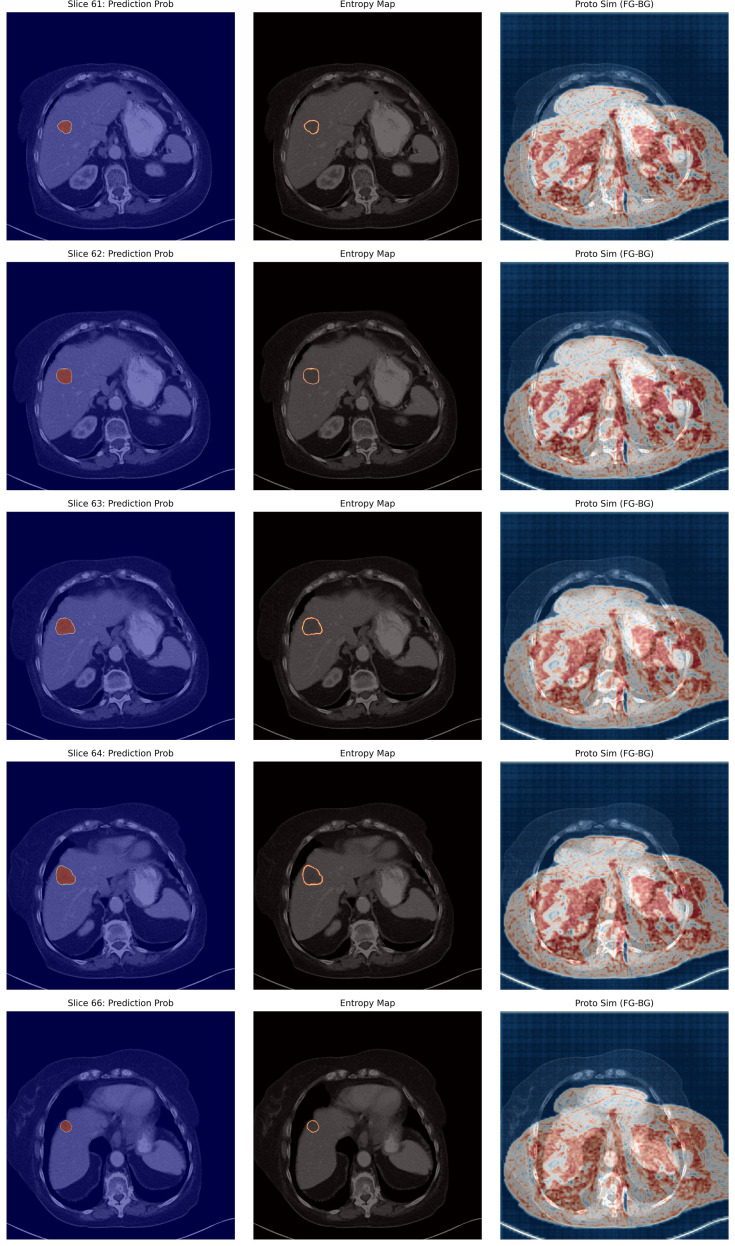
Entropy and prototype-response visualization for the same five slices shown in Figure 2. For each slice, the figure presents the CT image, the entropy map, the foreground prototype similarity map, and the background prototype similarity map. High-uncertainty responses are mainly concentrated around ambiguous lesion boundaries, while the foreground prototype similarity is concentrated in the tumor core and the background prototype similarity is stronger in surrounding non-tumor regions. This pattern suggests that the prototype memory provides discriminative guidance for resolving uncertain boundary regions rather than merely enlarging the foreground response.
